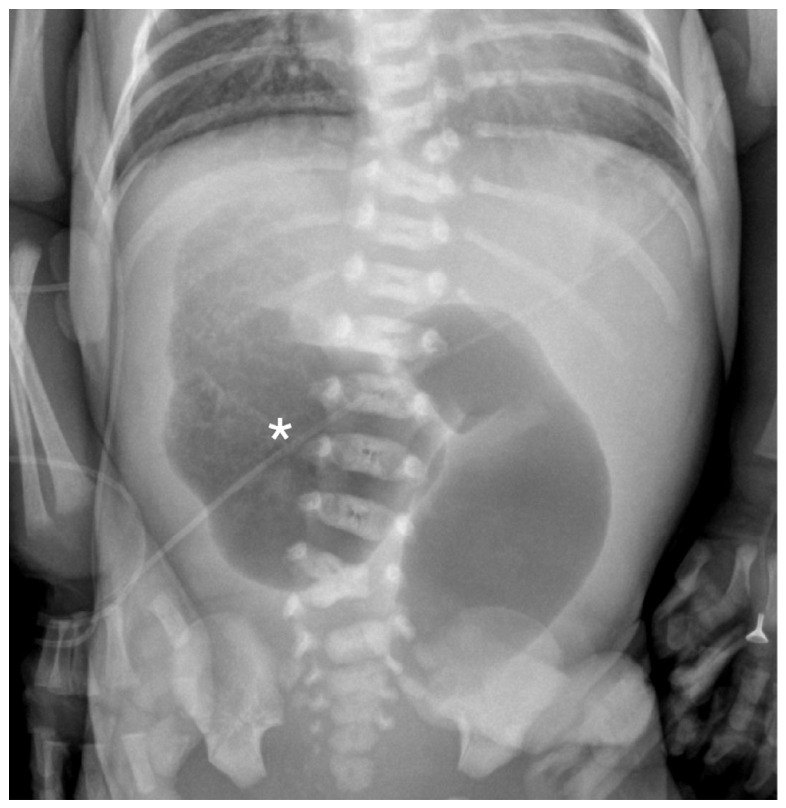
Abdominal radiograph of a female neonate with situs anomaly demonstrating a reversed double-bubble sign on the second day of life. The asterisk (*) indicates the right-sided stomach.
